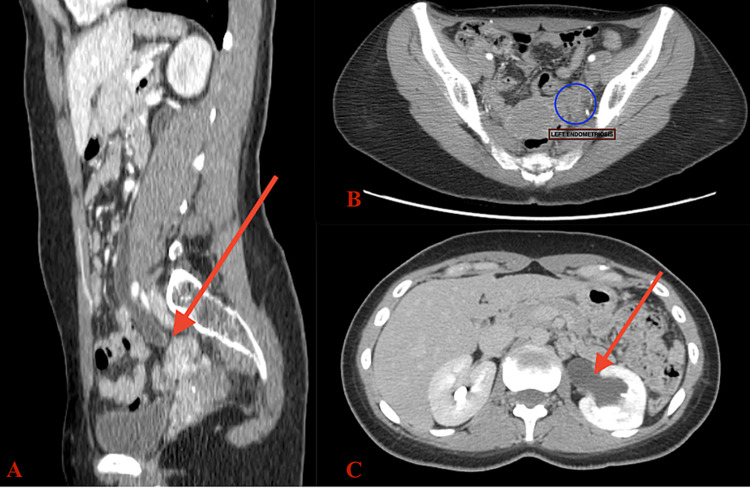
Contrast computed tomography (CT) scan showing (A) sagittal view of the abdomen with left hydroureter extending to mass (arrow), (B) axial view of left endometriotic mass (circle) and (C) axial view at the level of kidney demonstrating dilated left renal pelvis and severe left hydronephrosis.
